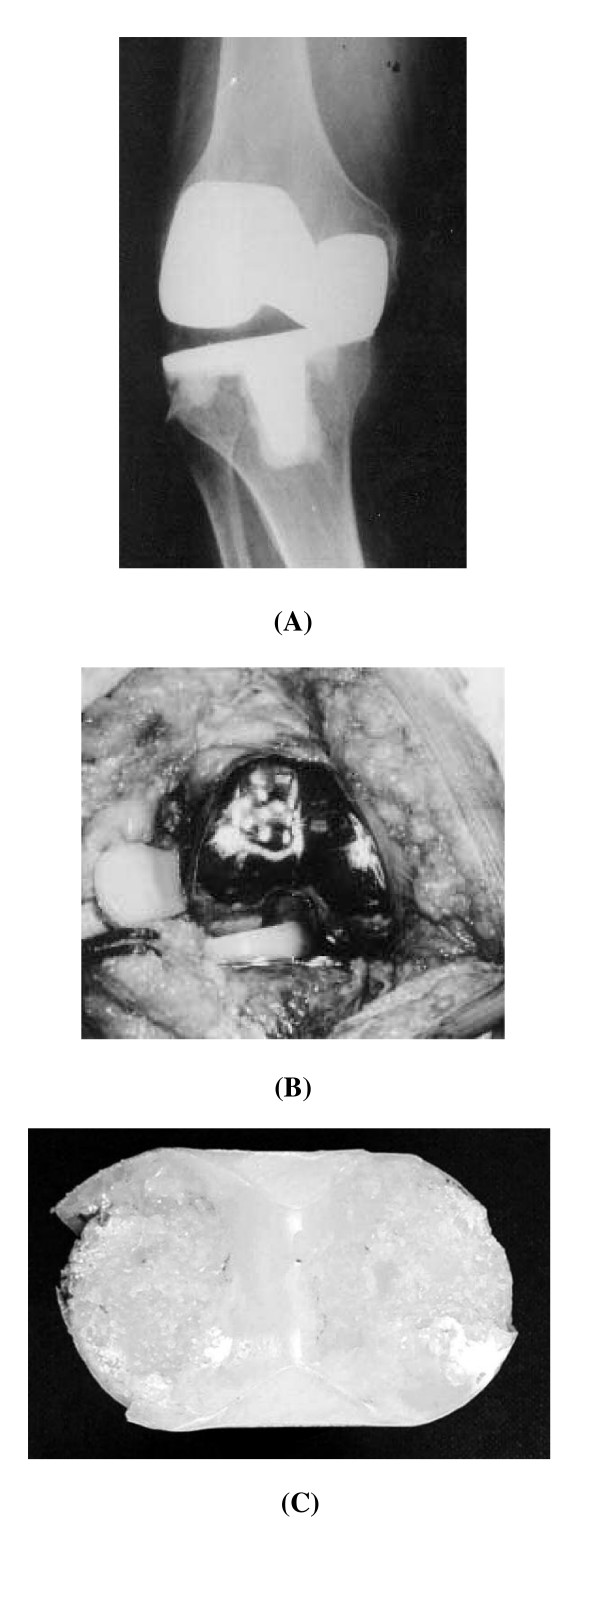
(A) An anteroposterior radiograph revealed complete absence of the femorotibial joint space. (B) At revision, the rotating platform was entrapped in the notch of the femoral component with 90° of rotation. (C) Wearing of the rotating platform is shown.
